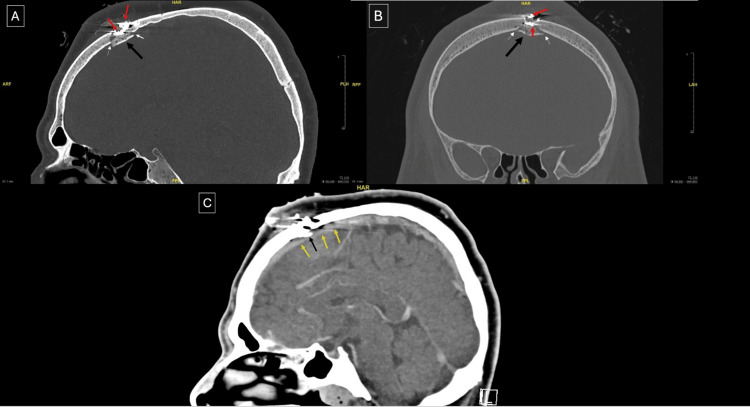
Preoperative non-contrast head CT demonstrating a bony island formed by the fracture (black arrow), with fracture margins indicated by white arrows. Red arrows denote bullet fragments adherent to the bony island. Yellow arrows indicate the SSS in close proximity to the bony island. (A) Sagittal section, bone window. (B) Coronal section, bone window. (C) Sagittal section, brain window CT: computed tomography, SSS: superior sagittal sinus
